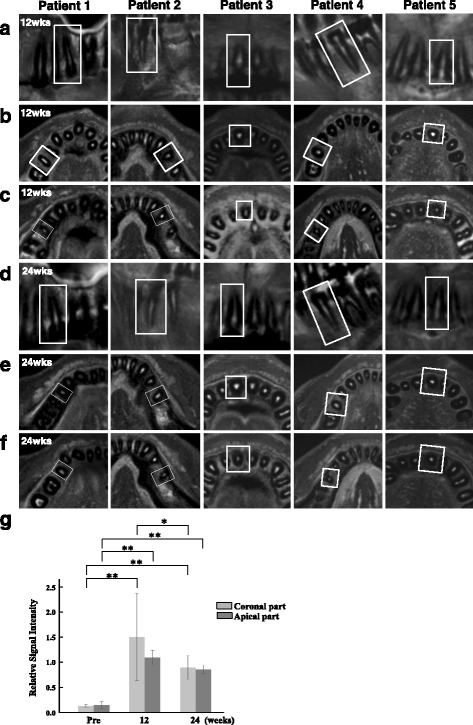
The changes of fat-suppressed T2-weighted (FST2W) MRI in cell-transplanted root canal in five patients. *Squares* indicate the teeth with cell transplantation. a–c Twelve weeks and d–f 24 weeks after transplantation of MDPSCs and G-CSF with collagen. a,d Sagittal slice; b,e axial slice at the coronal part of the root canal; c,f axial slice at the apical part of the root canal. g The relative SI of MRI. There are significant differences in the relative SI between pulpectomized root canal and cell transplanted root canal at 12 and 24 weeks both in the coronal part and apical part (*P* < 0.01); data are expressed as mean ± SD (*n* = 3). There is a significant difference in the relative SI of root canal between 12 weeks and 24 weeks in the coronal part (P* <0.05); data are expressed as mean ± SD (*n* = 4)
